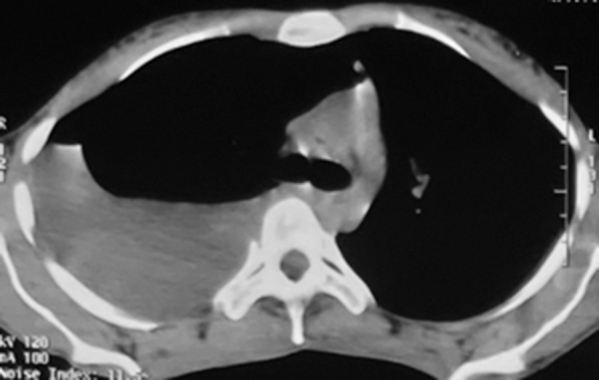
CT scan of the chest shows a hypodense opacity on the right hemithorax.
